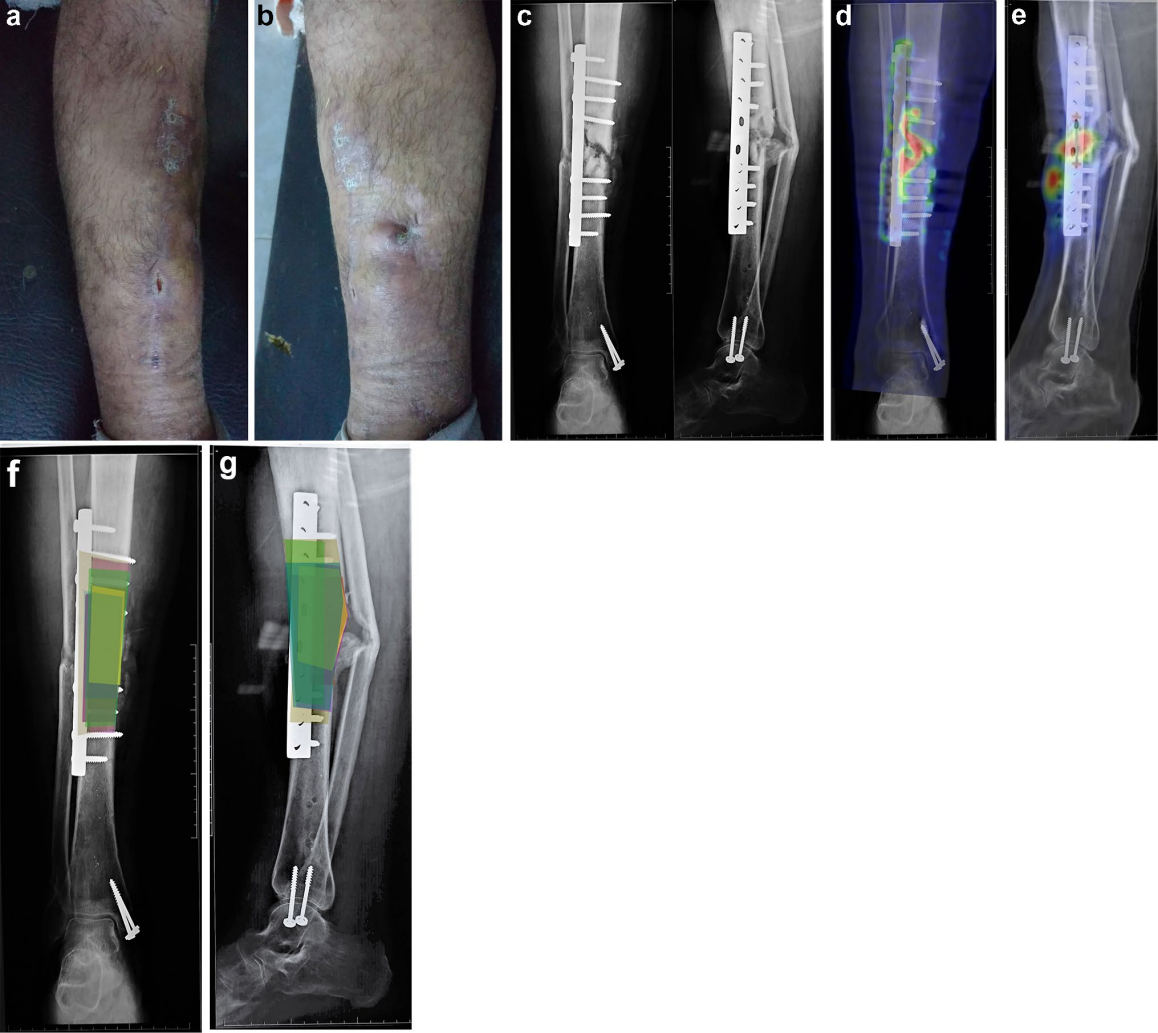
a Male patients, 30 years old, presented with postoperative osteomyelitis after fixation of Open fracture tibia after 9 months of infection development. The anterior aspect of the tibia shows a sinus. b Medial aspect of the leg shows a sinus. c Anteroposterior and lateral x-rays showed an infected non-united fractured tibia. d  18F-FDG PET-CT image merged with AP view of the x-ray, shows the clear demarkation of infection with the red colour referring to the highest uptake. e PET-CT image merged with lateral view of the x-ray, shows the clear demarkation of infection with the red colour referring to the highest uptake. f and g Anteroposterior and lateral x-ray with the layers drawn by the ten consultants, variability of the length can be appreciated
